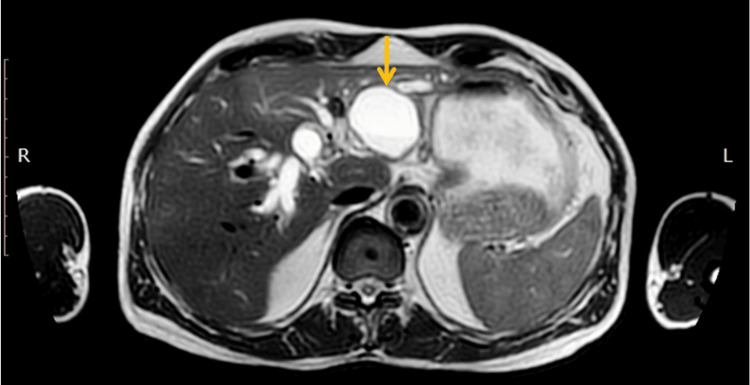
T2 axial section of MRI abdomen. Cystic lesion (arrow) in segment II-III with bilateral intrahepatic biliary duct dilatation.
